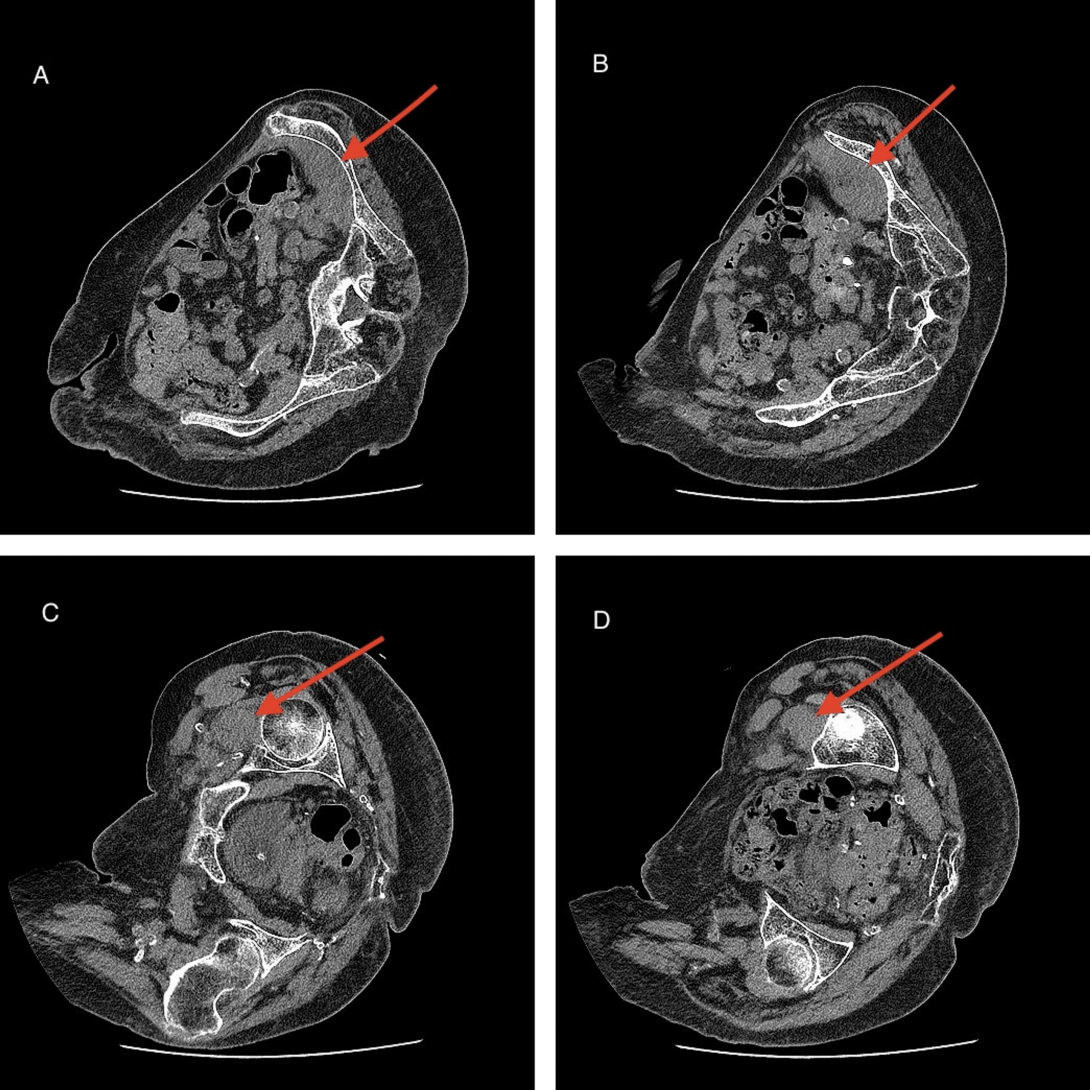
CT pelvis showing spontaneous iliopsoas tendon rupture (A) Axial CT at the level of the iliac crest showing bulky soft tissue along the left iliacus muscle (arrow).
